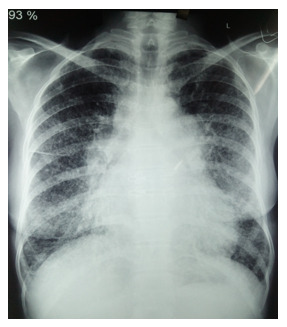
Chest X-ray showing bilateral reticulo-nodular infiltrates involving the middle and lower zones and some parts of the upper zones.
